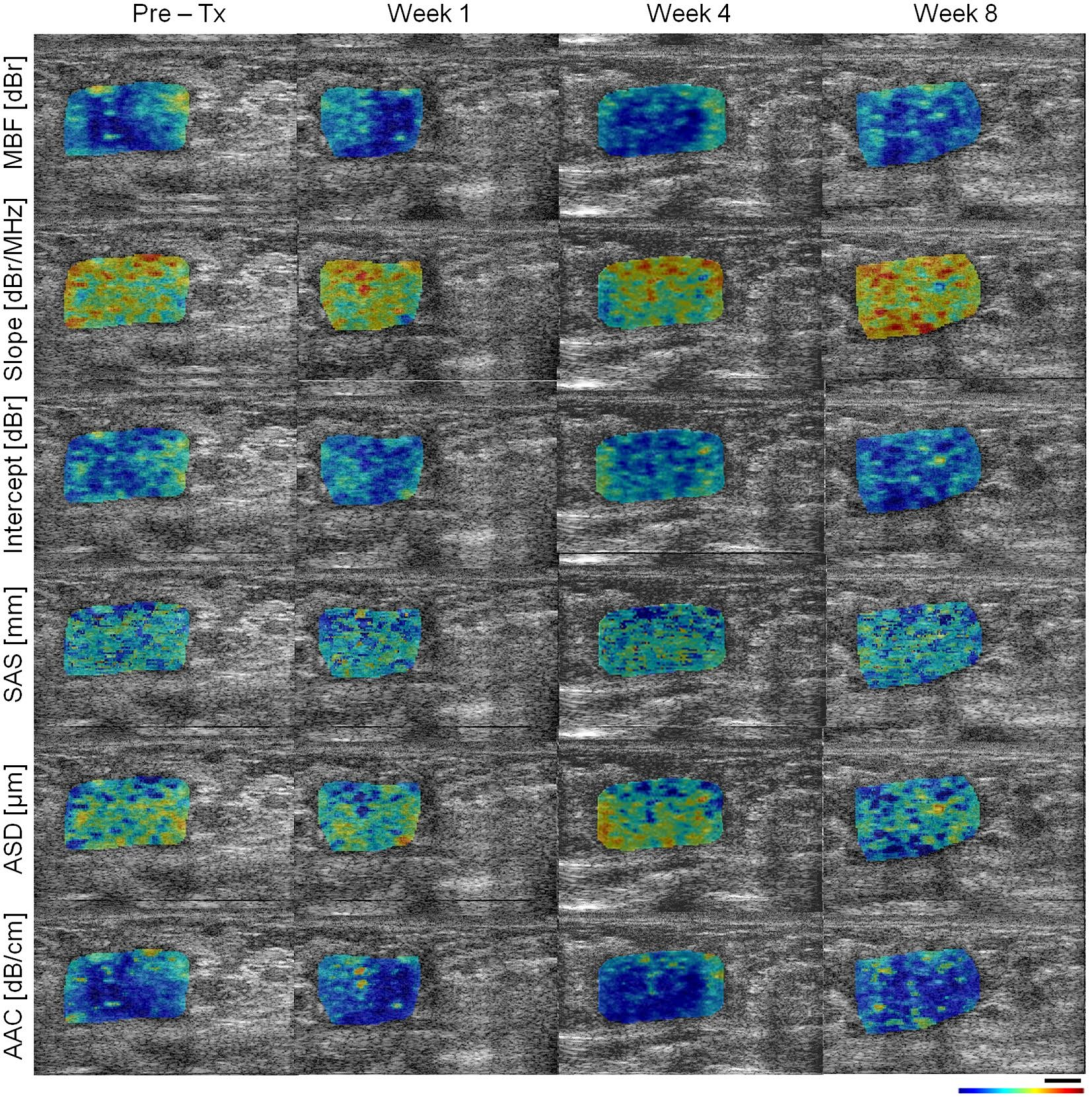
Representative ultrasound B-mode images with QUS parametric overlays (described in Fig. 1) acquired from a non-responding patient prior to and at different times after the start of chemotherapy. The color bar represents a scale encompassing 35 dBr for MBF, 10 dBr/MHz for slope, 65 dBr for intercept, 3 mm for SAS, 150 µm for ASD, and 55 dB/cm^3^ for AAC. The scale bar represents 5 mm.
